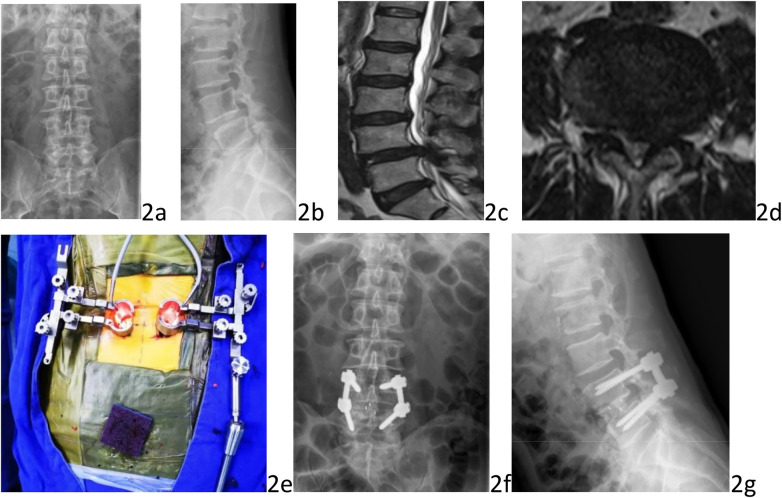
A 72-year-old male was diagnosed for lumbar disc herniation with preoperative, intraoperative, and postoperative images of bilateral channel MIS-TLIF. Preoperative anteroposterior and lateral radiographs showed lumbar instability (a,b). Preoperative lumbar MRI showing L4/5 disc herniation with instability (c,d). Intraoperative bilateral channel procedure (e). Postoperative anteroposterior and lateral lumbar radiographs demonstrating satisfactory position of internal fixation (f,g).
